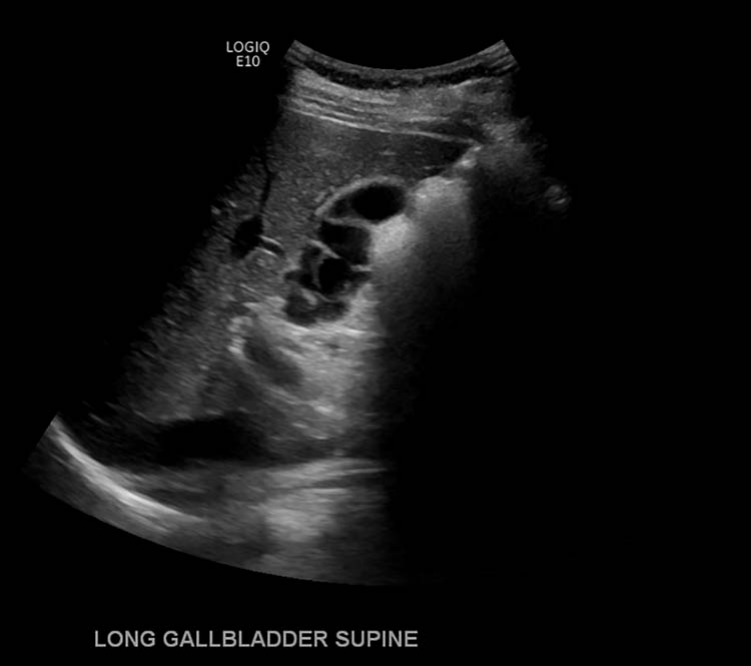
Ultrasound imaging of the gallbladder showing multiple septations resulting in a ‘sack of grapes’ appearance.
